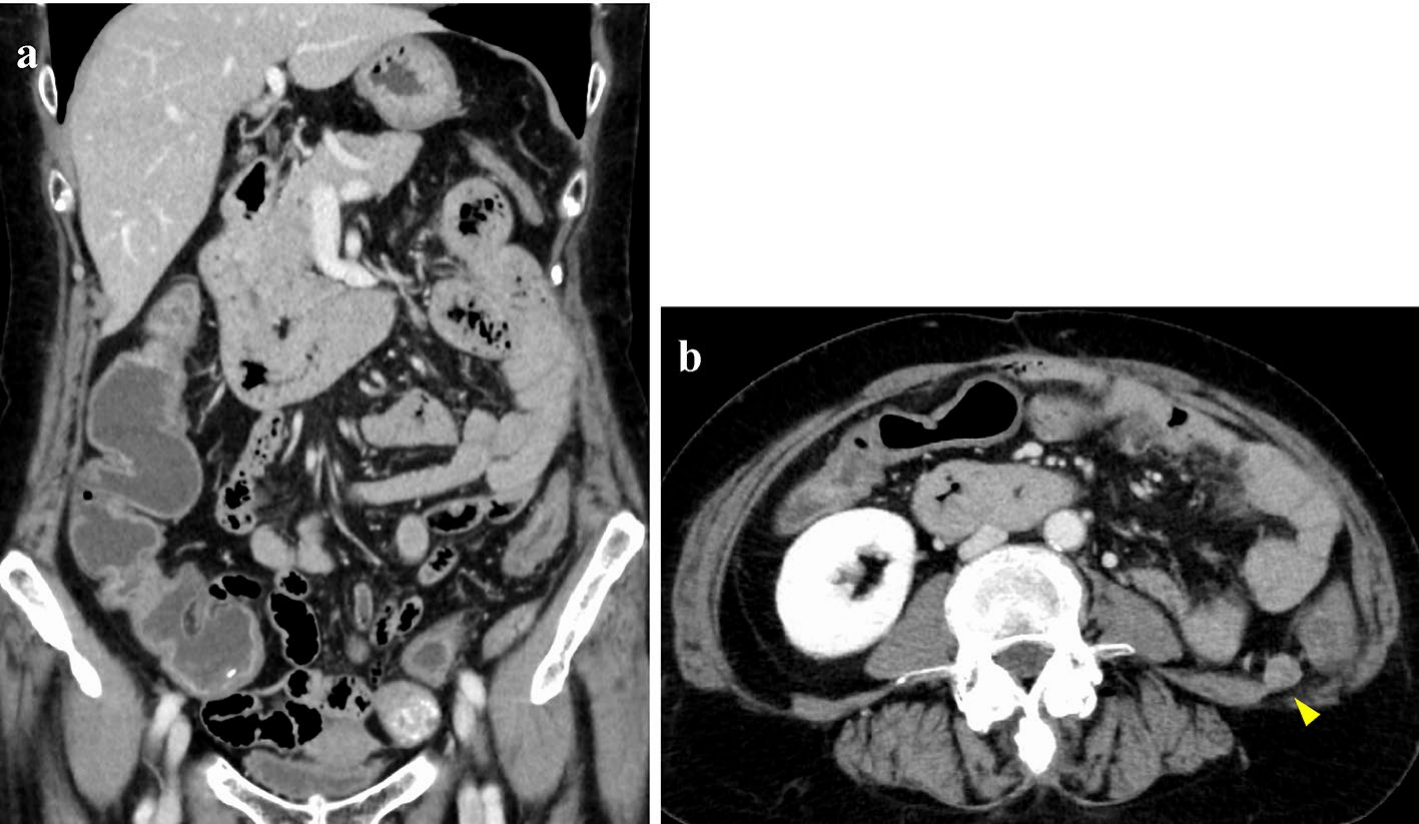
Contrast-enhanced abdominal computed tomography (CT) when diagnosed with pazopanib-induced enteritis. (a) Coronal section showing diffuse wall thickening of the colon. (b) Horizontal section showing metastases of the renal cancer in the left retroperitoneal cavity (arrowhead)
